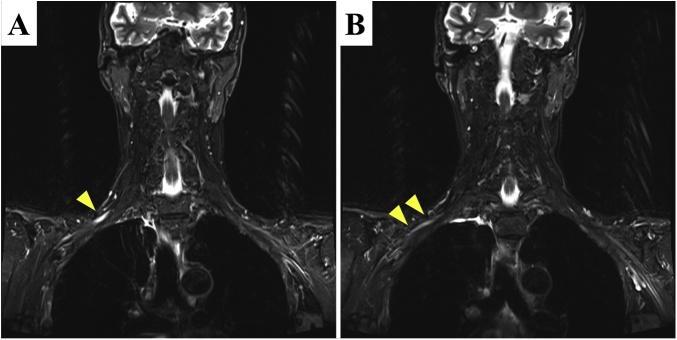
Findings of short-tau inversion recovery sequences in MRI. Short-tau inversion recovery sequences in MRI showed (A) clear high signal intensity and edematous swelling of the right posterior cord of the brachial plexus (arrowhead) at the costoclavicular space and (B) slightly high signal intensity (arrowheads) on the distal side of the cord. These signals indicated the most severely damaged part.
